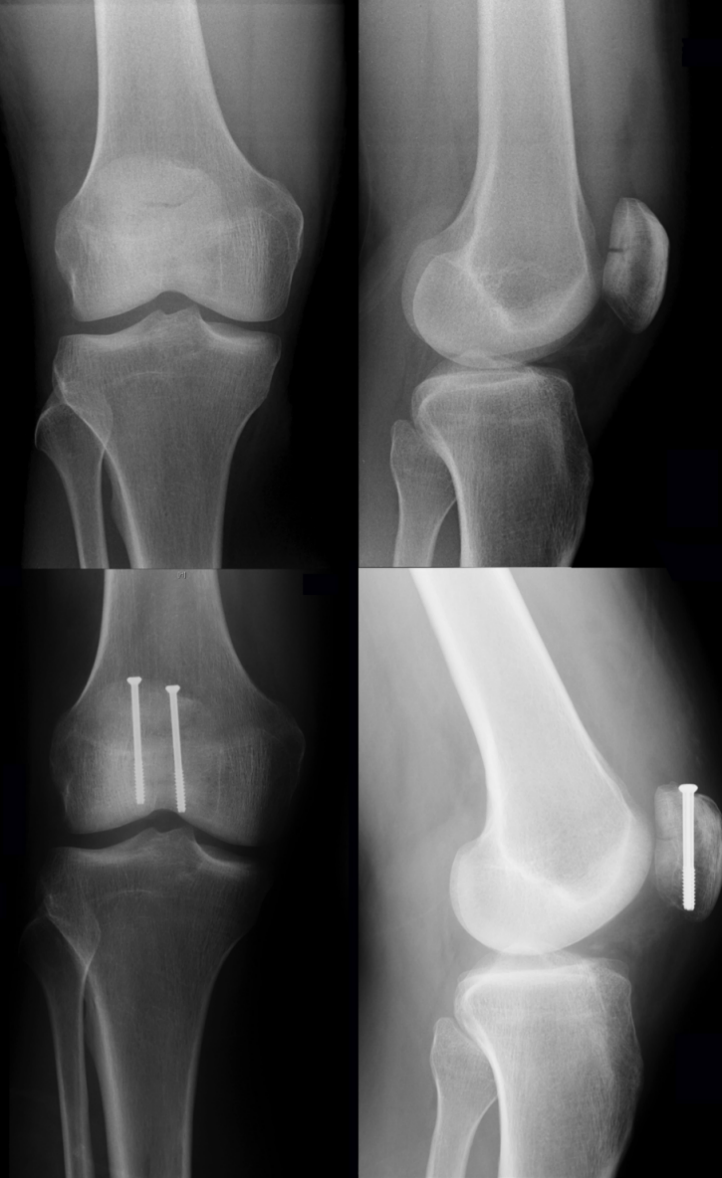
Preoperative X-rays of a non-comminuted transverse patella fracture (above). 3 months post-surgery using percutaneous screw fixation (below).
